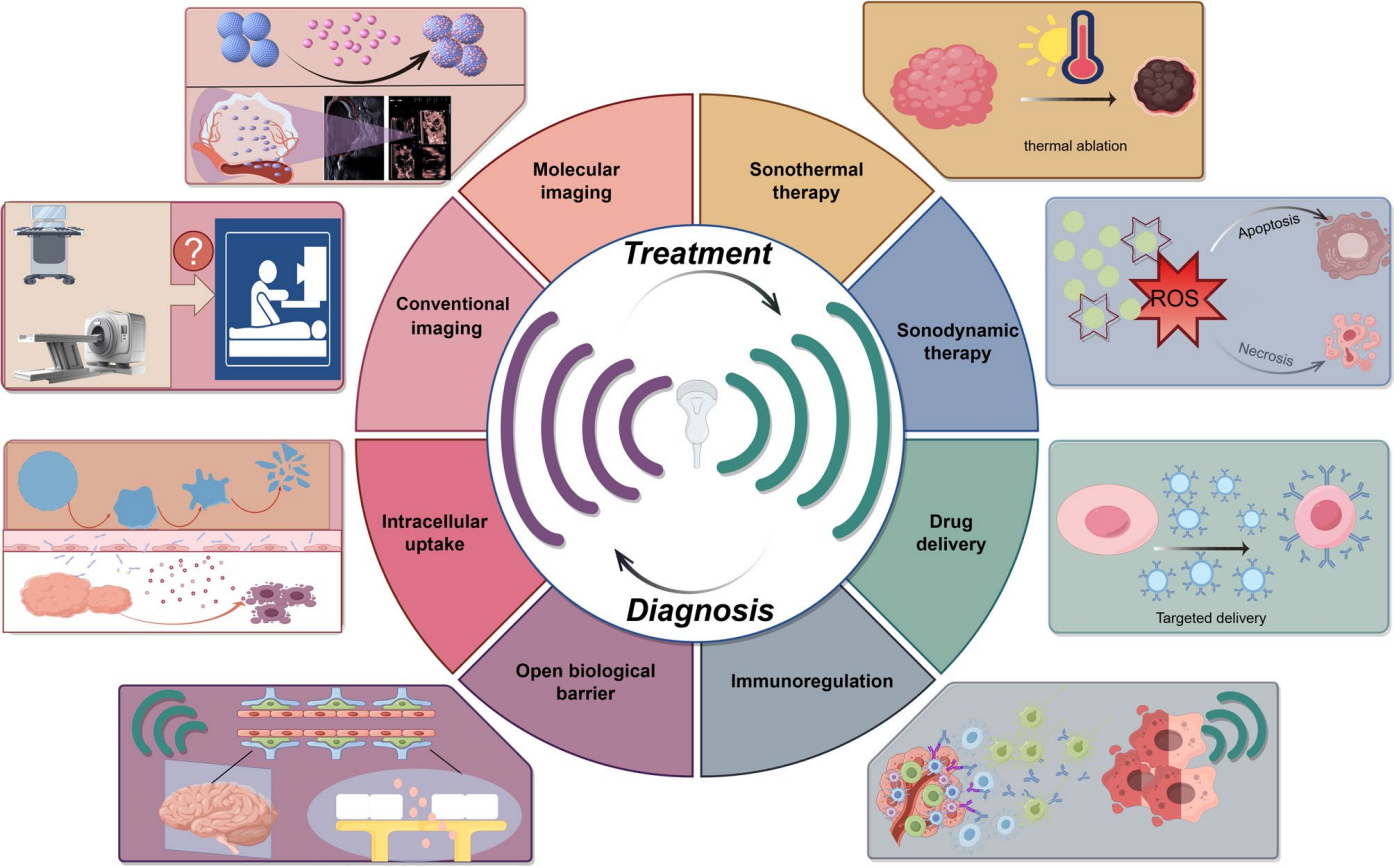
Role of Ultrasound in Tumor Management
